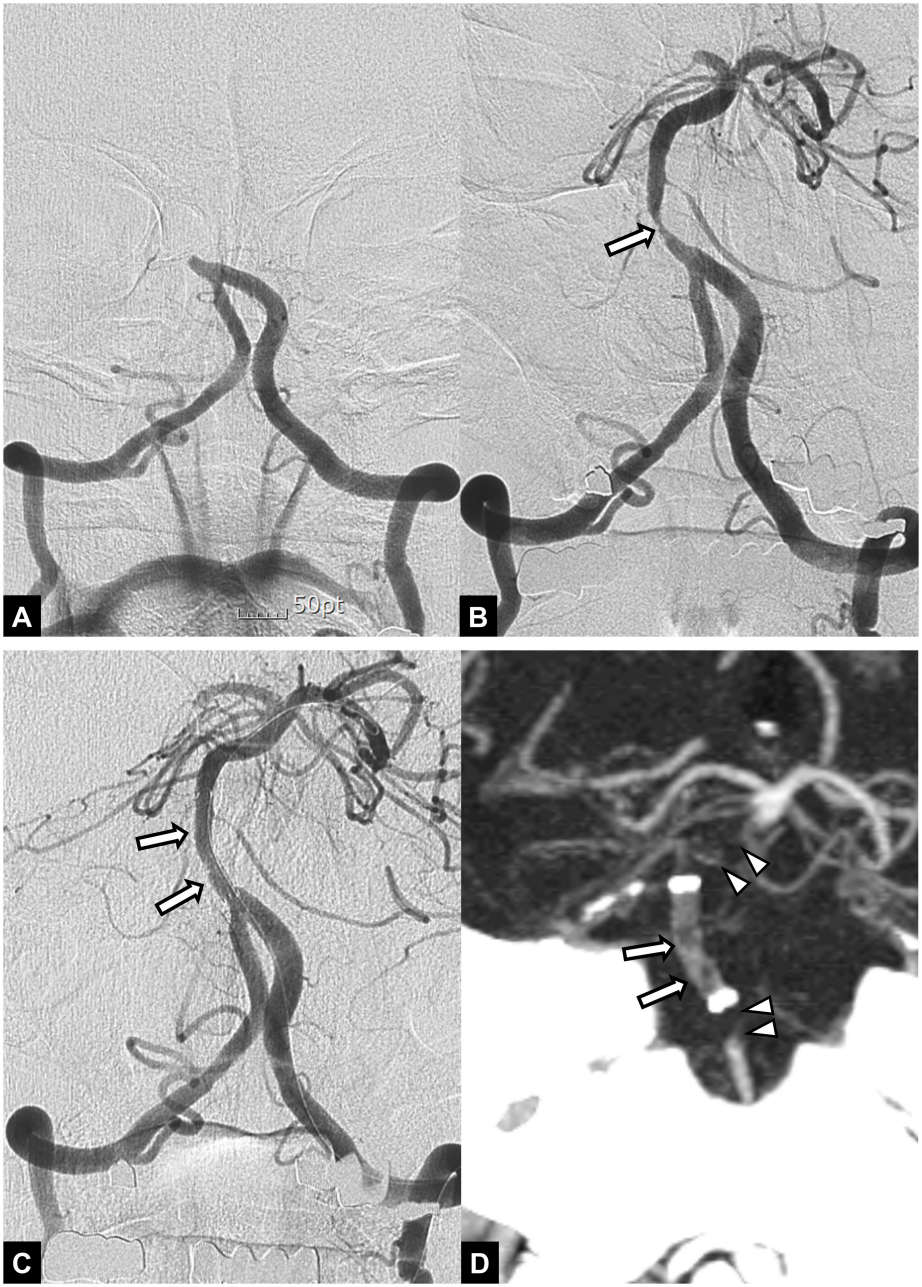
A 55-year-old male patient with acute stroke due to basilar artery occlusion. (A) Initial angiogram shows an occlusion at the proximal segment of the basilar artery. (B) Angiogram obtained after mechanical thrombectomy with a stent retriever shows recanalization of the basilar artery with underlying severe stenosis (arrow). Note accompanying occlusion in the right posterior cerebral artery. (C) Final angiogram obtained after angioplasty and stent implantation (arrows) to treat underlying stenosis shows successful recanalization of the basilar artery. (D) Follow-up CT angiography obtained 24 h after endovascular treatment shows occlusions in the implanted stent (arrows) and proximal and distal segment of the basilar artery (arrowheads). The patient died 2 days later.
